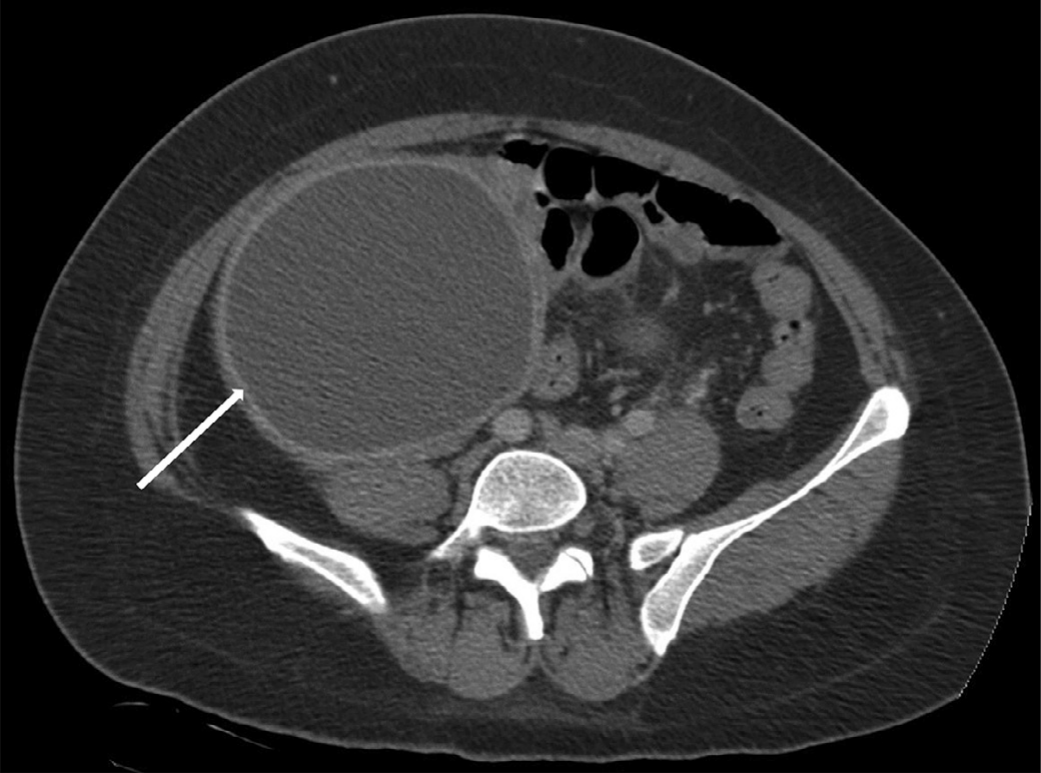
NECT of the pelvis demonstrates a large fluid containing structure in the right adnexa (white arrow) that corresponds to the pelvic ultrasound (Fig. 1) that was compatible with an endometrioma.
